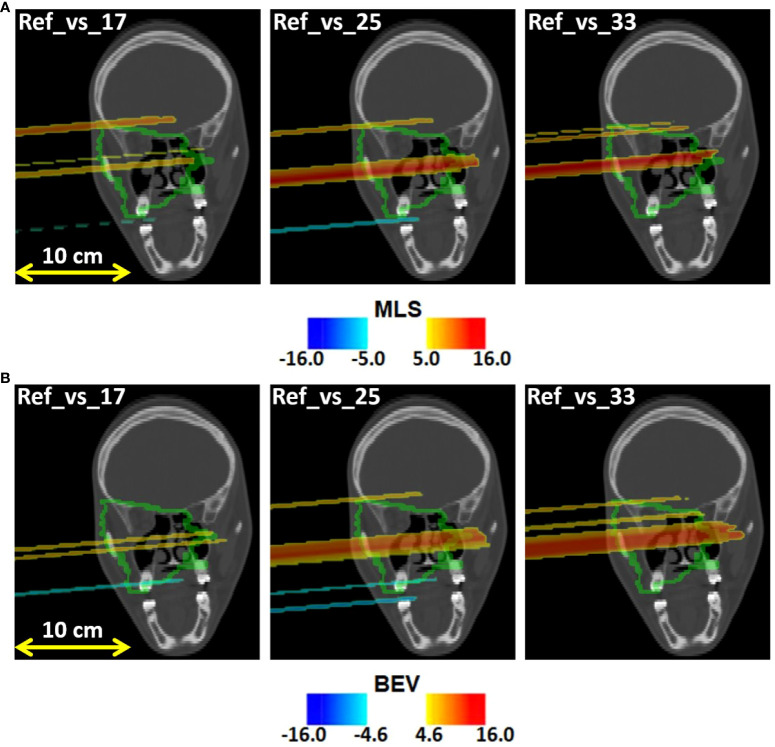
Coronal views of the control CT of patient 006P, with the (A) MLS outliers map *O_MLS_
